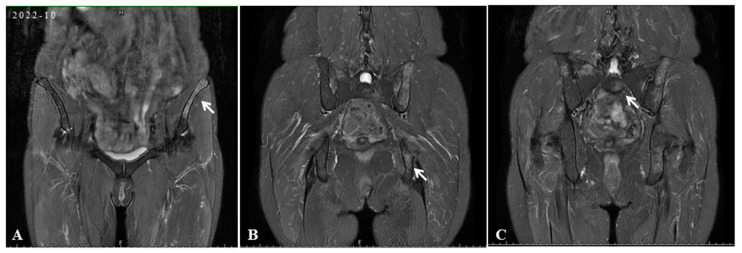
In the initial scan, pelvis MRI identified irregular patchy abnormal signals in the ilium (white arrow) (A), left ischium (white arrow) (B), and sacrum (white arrow) (C).
